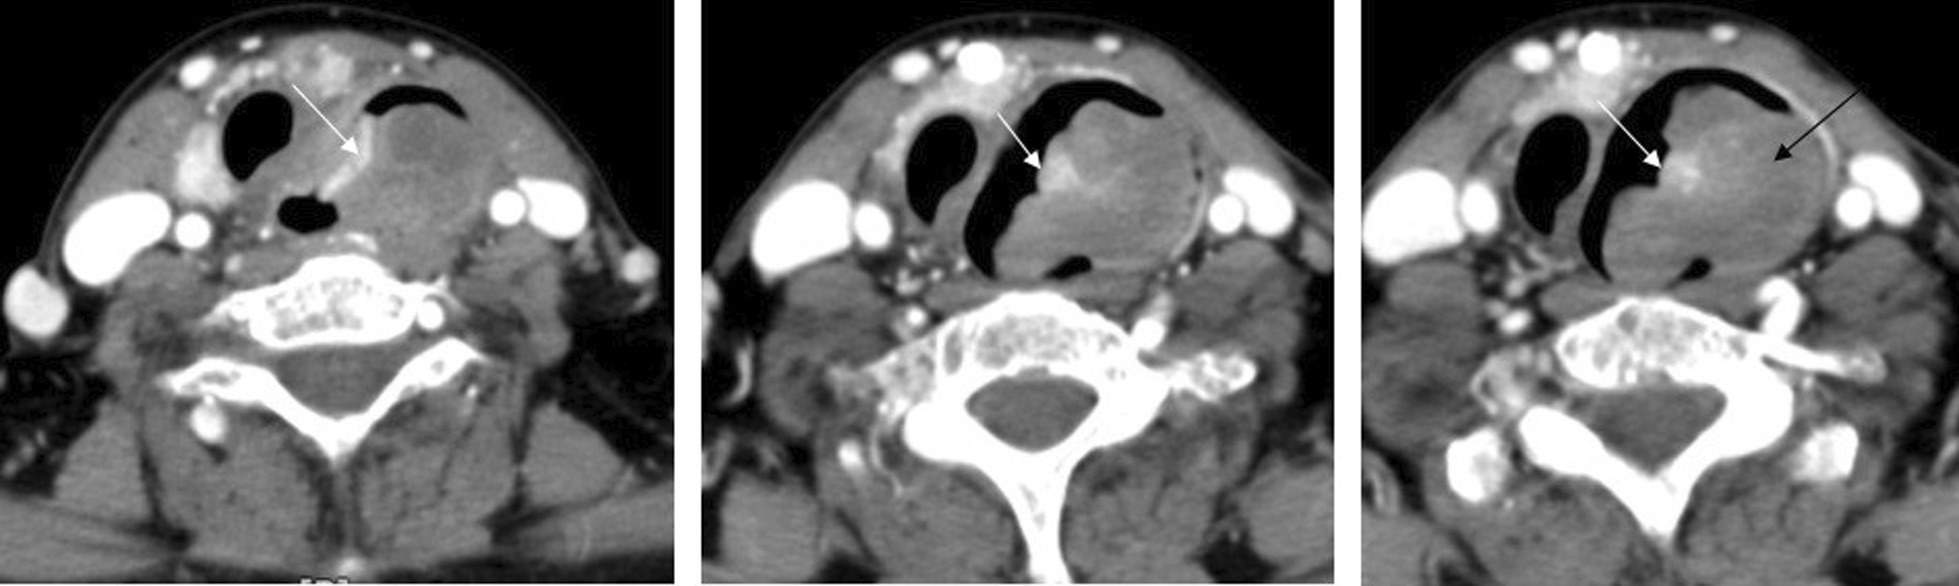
Post-contrast computed tomography images showing two lesions (hugging lesions) in the upper esophagus causing the tracheal shift to the right side: larger lesion (black arrow) is isodense with an area of the necrosis hugging the other small hyperdense lesion (white arrows) arising from the other side of the lumen
